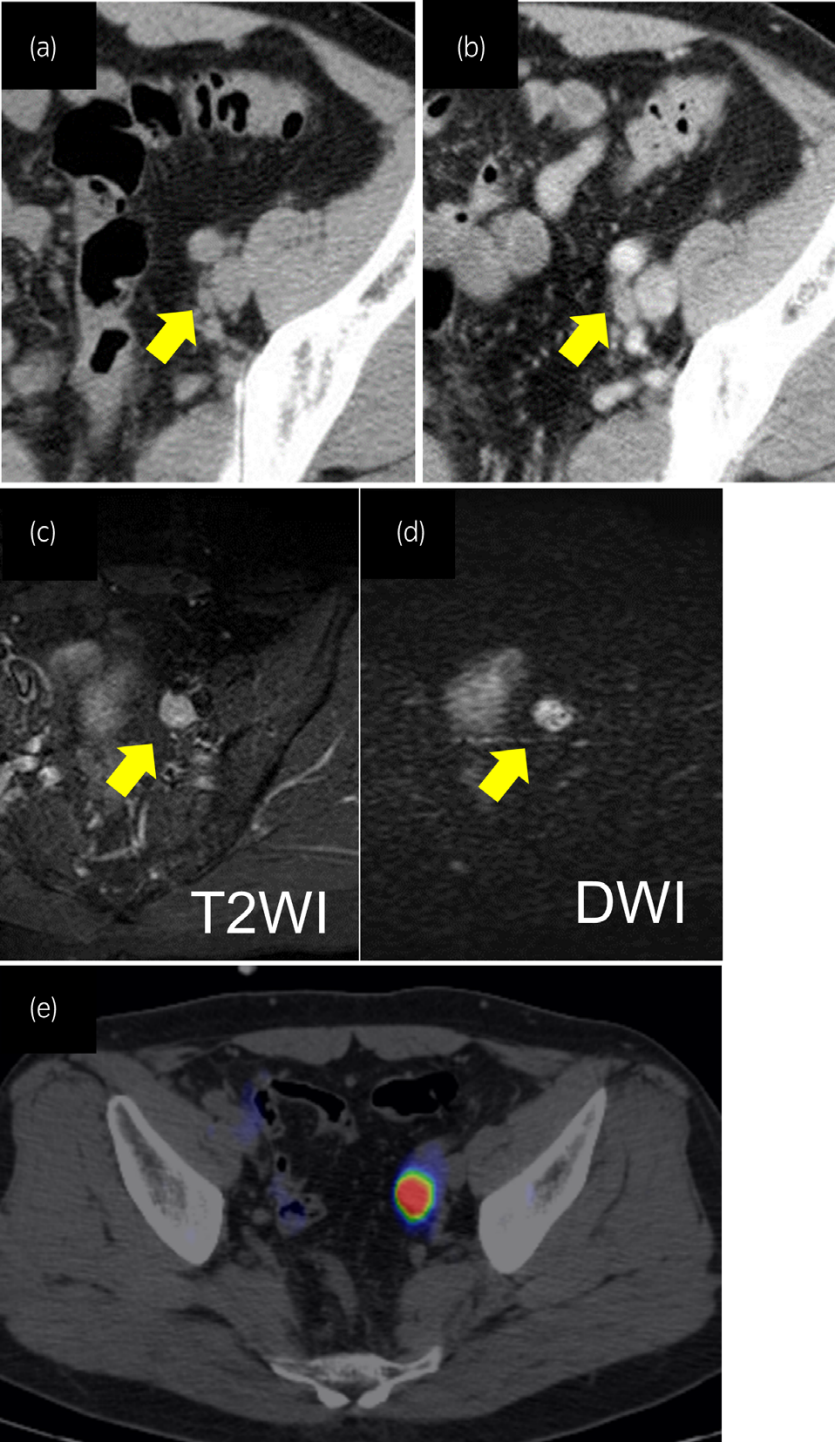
Imaging findings of left external LN swelling in a patient with testicular seminoma after left orchiectomy. LN swelling at (a) 7 years and (b) 9 years after orchiectomy. The lesion had a (c) high T2 image signal intensity and (d) high diffusion‐weighted imaging signal intensity. (e) Positron emission tomography/computed tomography fusion imaging revealed the accumulation of 18F‐labeled fluorodeoxyglucose in the left external LN.
